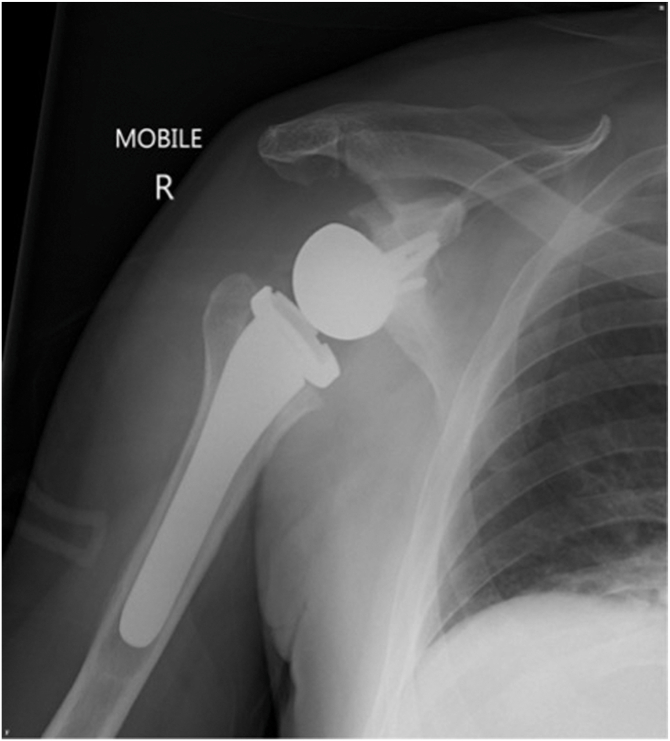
Immediate postoperative radiographs, anteroposterior view.
